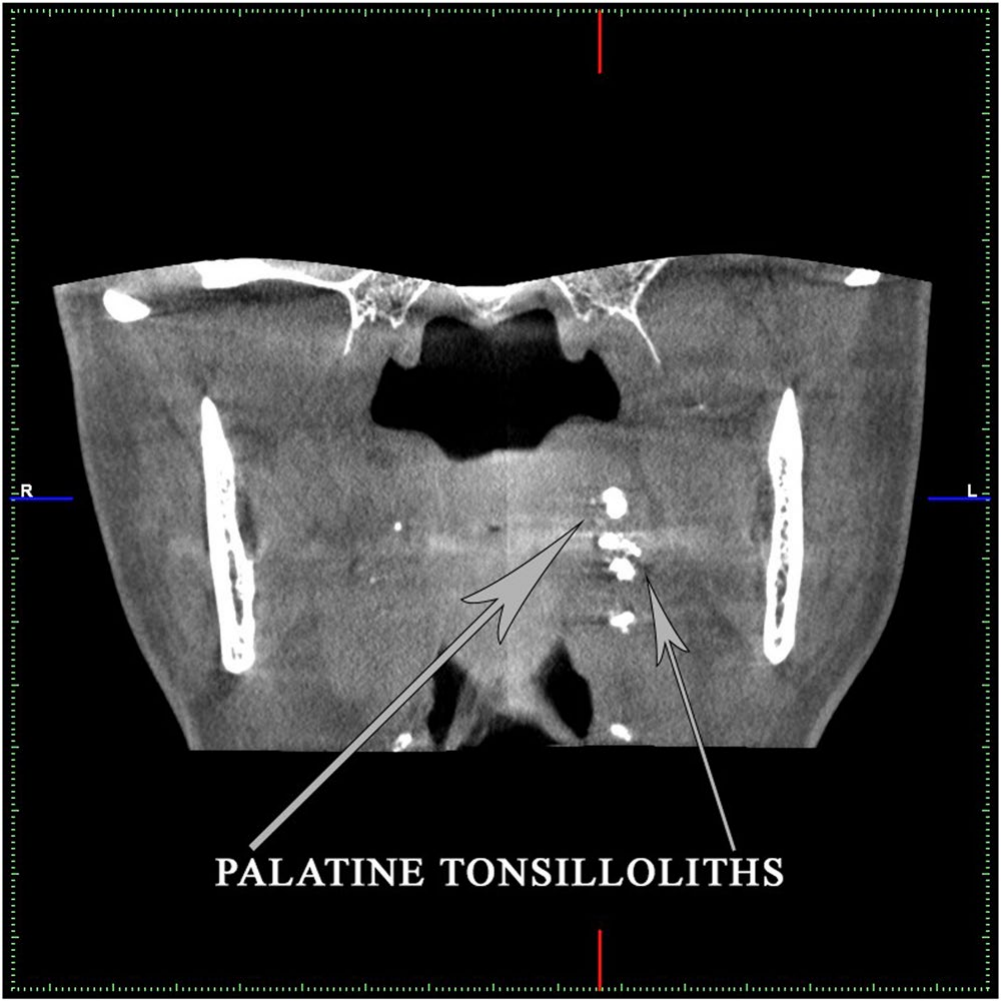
CT image of a patient diagnosed with PT. One can see multiple PT.
